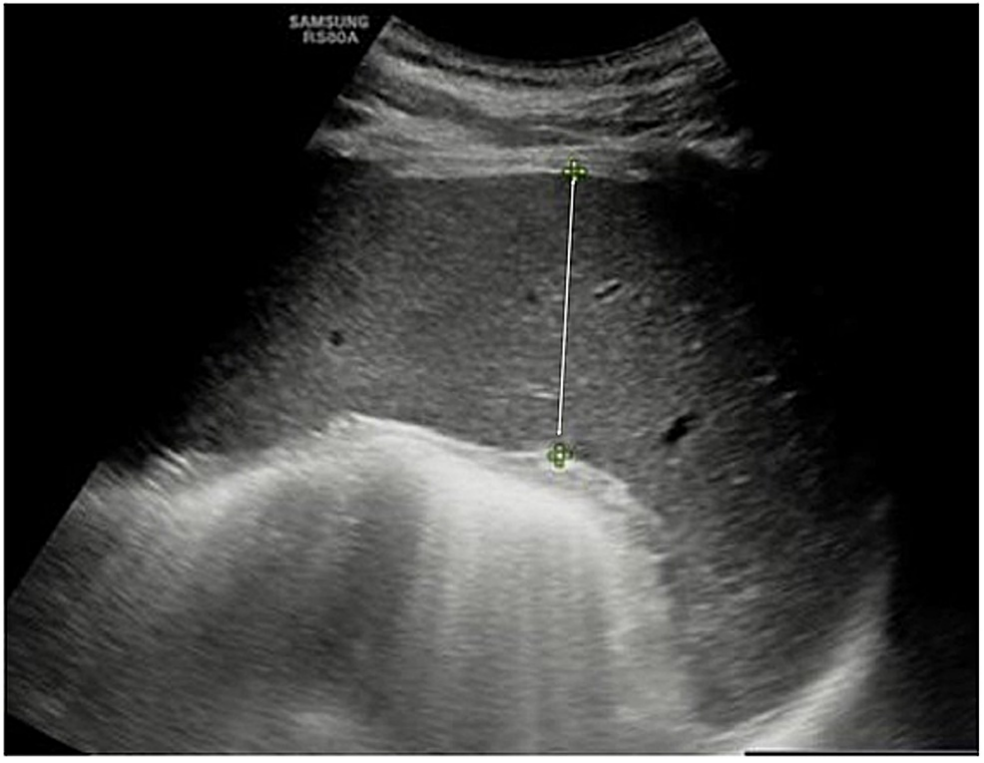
Ultrasound showing splenomegaly.
